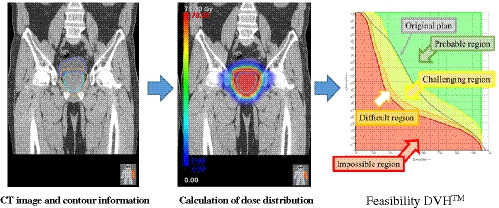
Feasibility DVH^TM^ calculation method. Computed tomography images, contour information, and prescription dose for the target are defined, and the ideal dose distribution is calculated. Then, based on the calculated dose distribution and contour information, the feasible dose volume histogram (DVH) is calculated.
